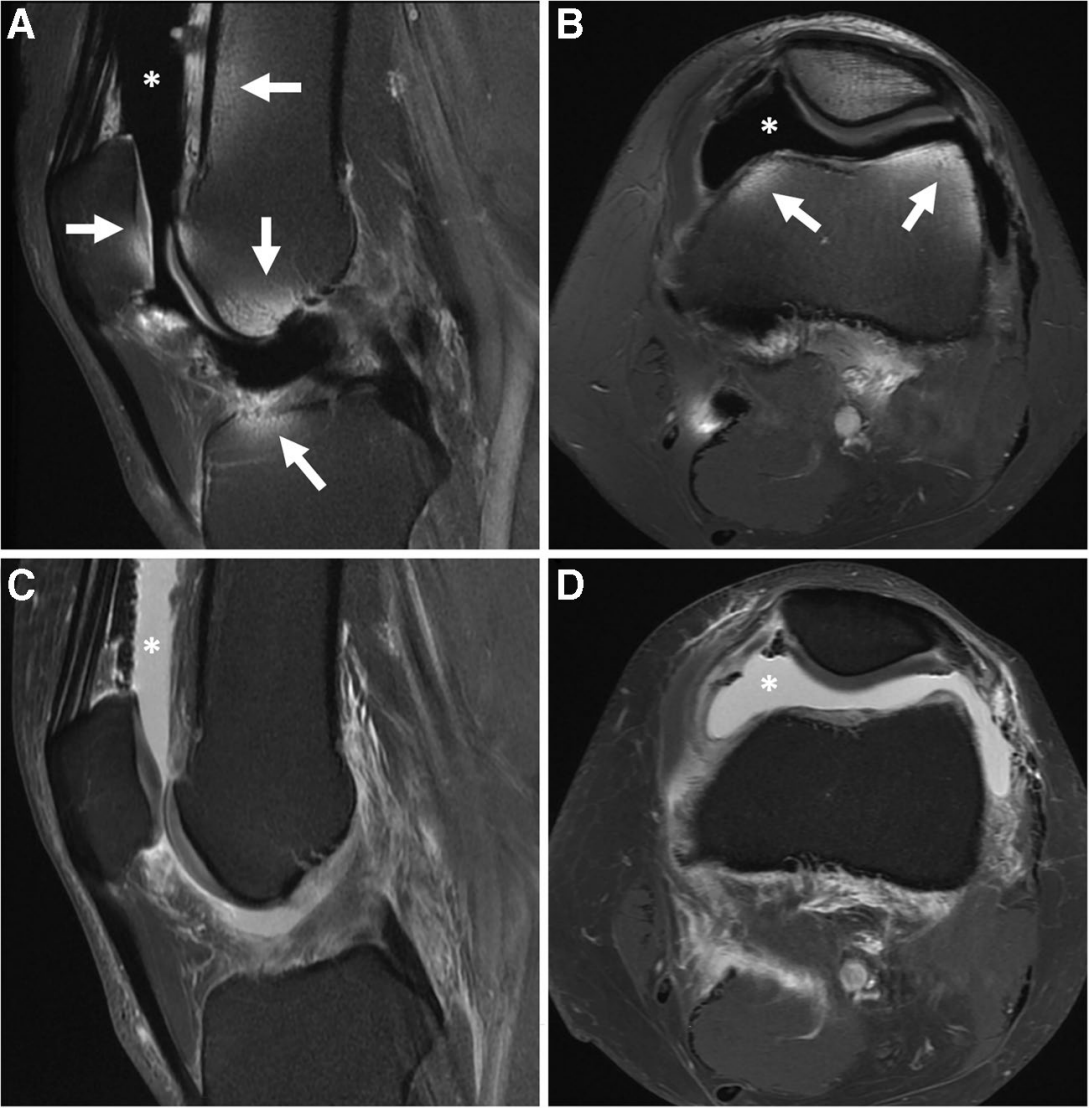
“Black” contrast effect. Thirty-four-year-old man with history of meniscus surgery and persistent knee pain. Sagittal (A) and axial (B) T1-weighted fat-suppressed 3 T MR images obtained immediately after inadvertent injection of a higher concentration of gadolinium contrast shows hypointense contrast (asterisks). Notice regions of inhomogeneous fat suppression due to the strong paramagnetism of concentrated gadolinium (arrows). Sagittal (C) and axial (D) T1-weighted fat-suppressed MR images after a 3.5-h delay shows that the majority of the injectate is now hyperintense (asterisks) and there is homogeneous fat suppression
